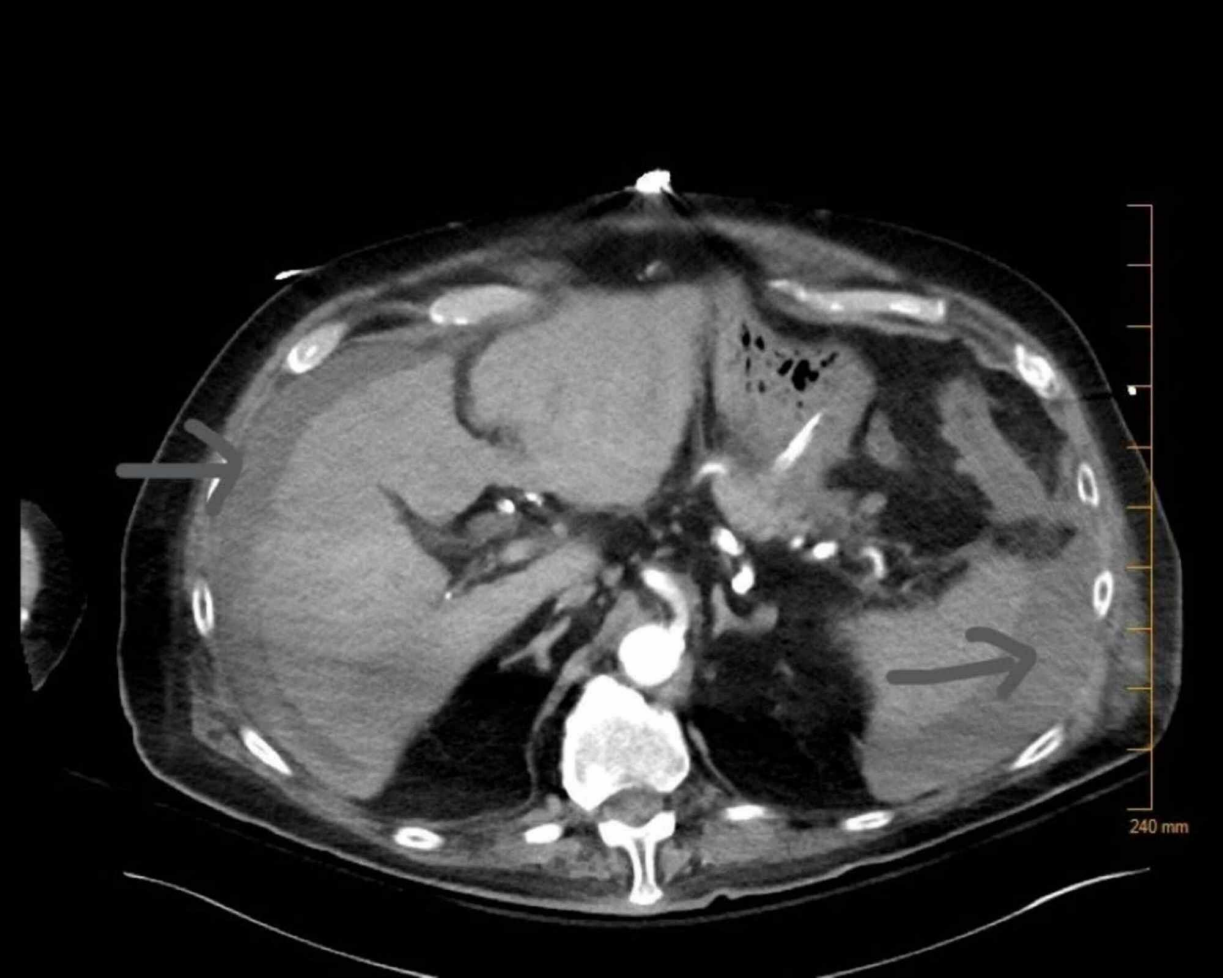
CT showing blood in the abdomen
